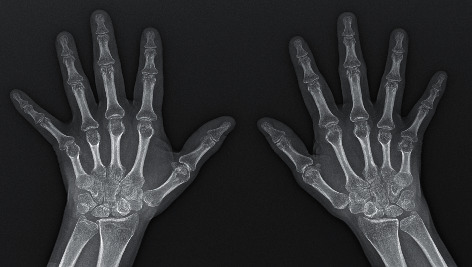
Plain radiographs of both hands.
